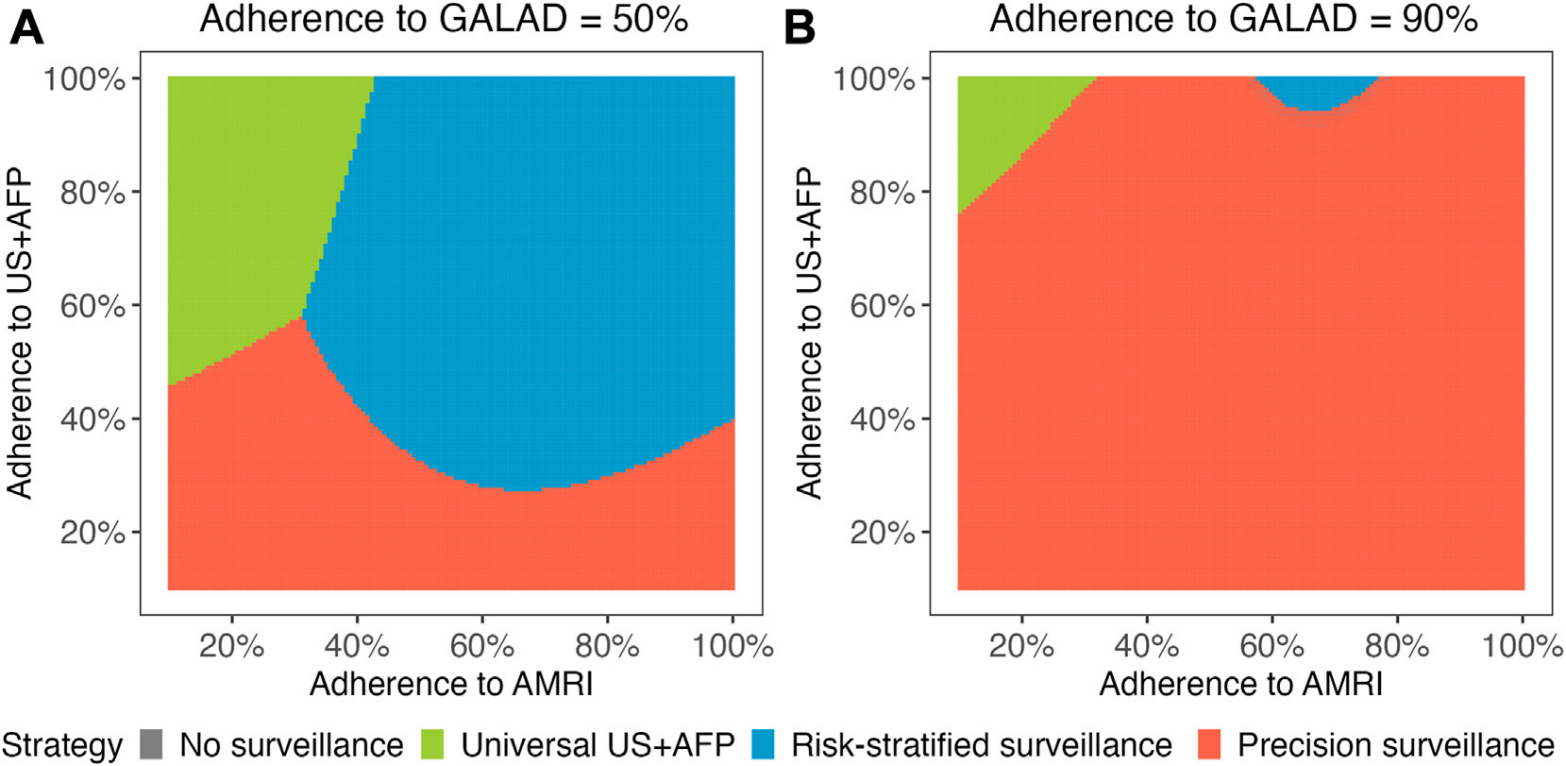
Three-way sensitivity analysis for adherence of surveillance modalities. The most cost-effective surveillance strategy was impacted by adherence to US + AFP, GALAD, and AMRI, albeit with non-linear associations. When the adherence for GALAD was 50%, precision surveillance outperformed the other surveillance strategies at lower adherence rates for US + AFP; however, universal US + AFP and risk-stratified surveillance were preferred when US + AFP and abbreviated MRI adherence increased. If adherence to GALAD was 90%, precision surveillance was the optimal strategy unless the adherence to US + AFP exceeded ∼80%. AFP, alpha fetoprotein; AMRI, abbreviated MRI; GALAD, Gender, Age, AFP-L3, AFP, and DCP; US, ultrasound.
